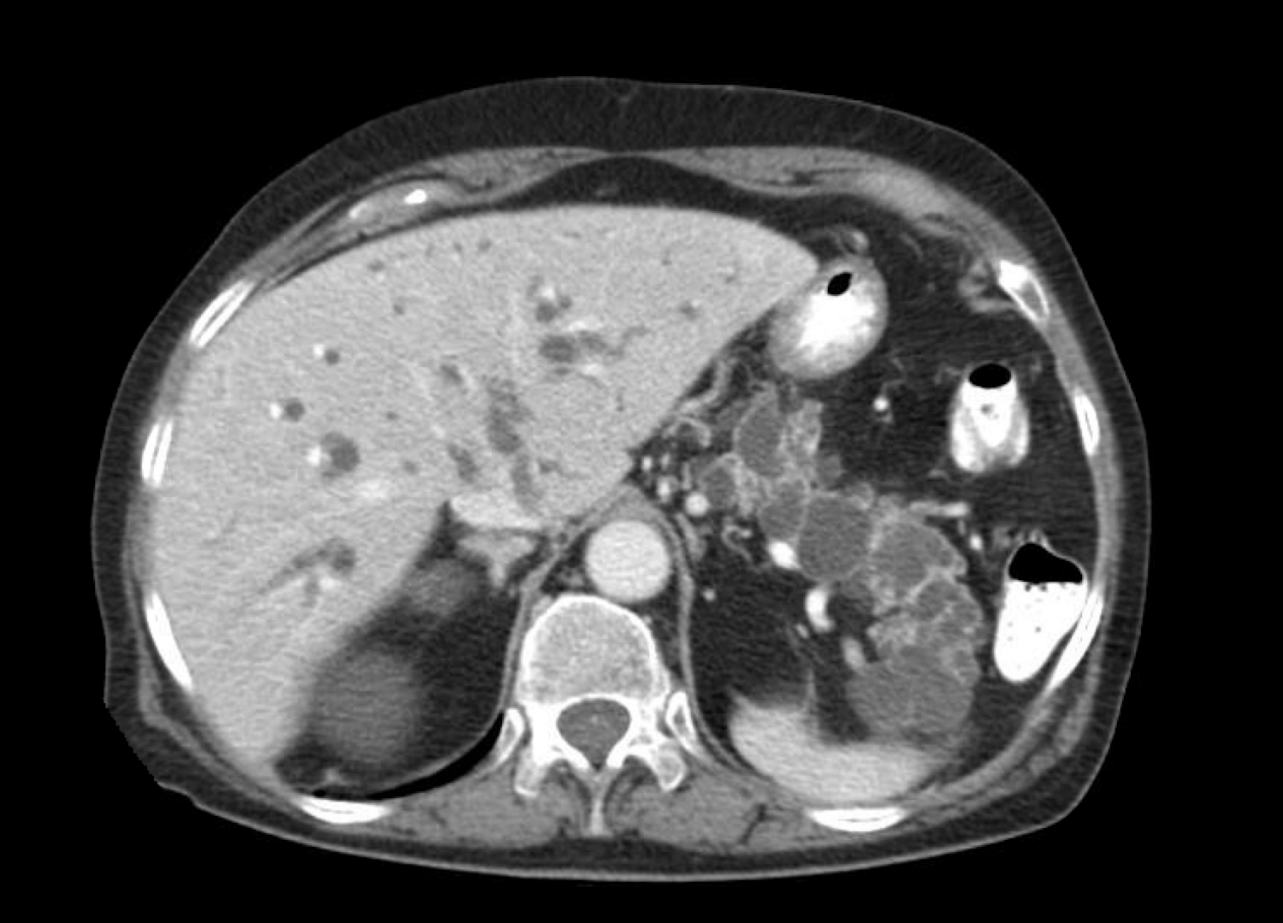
CT Abdomen with Contrast Contrast CT scan demonstrating numerous secondary cystic dilatations in the tail of the pancreas.
